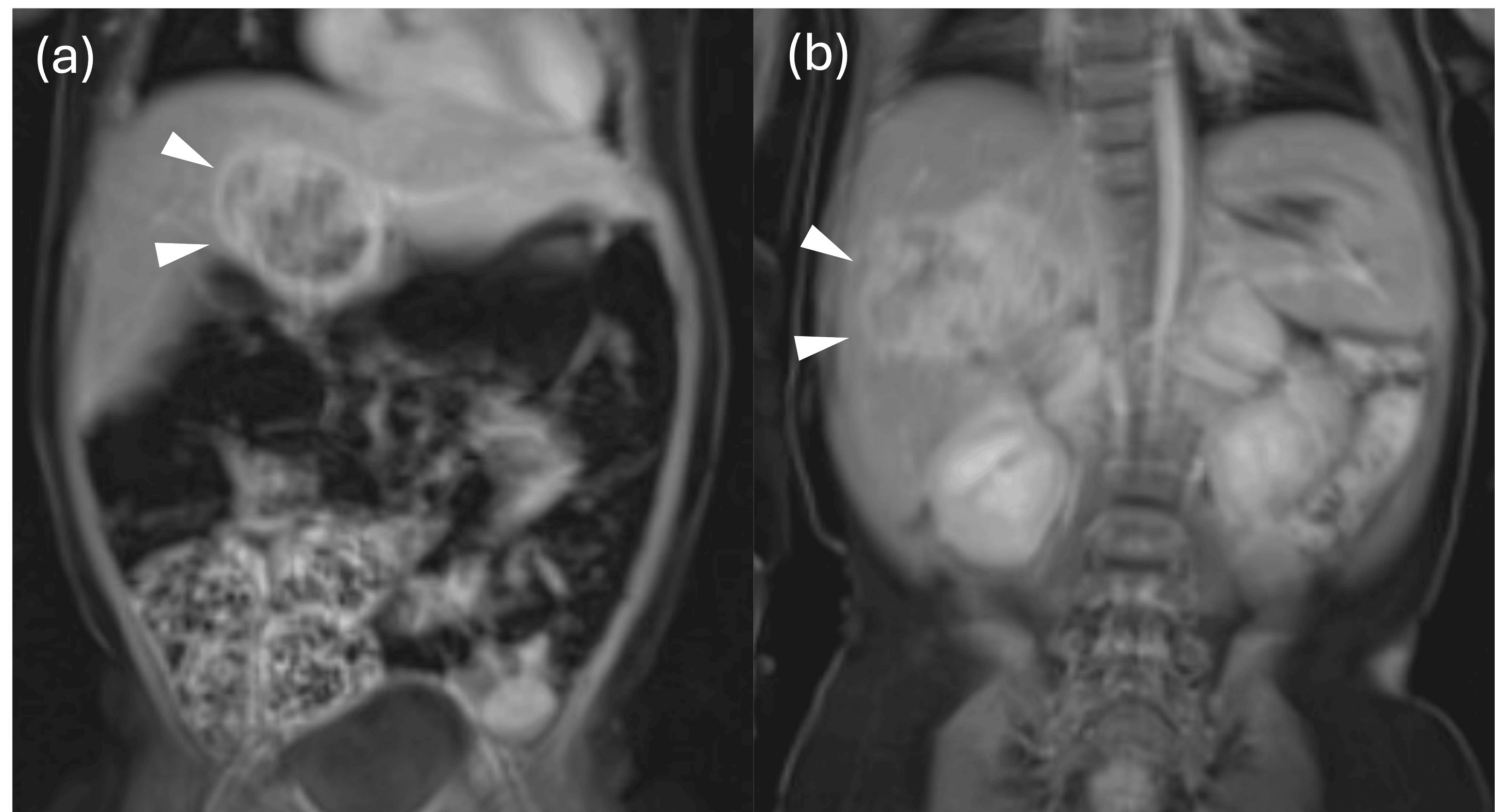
Magnetic resonance imaging (MRI) of the liver tumors (a) The segment 4 tumor and (b) the segment 6 tumor. The white arrowheads indicate the tumor.
